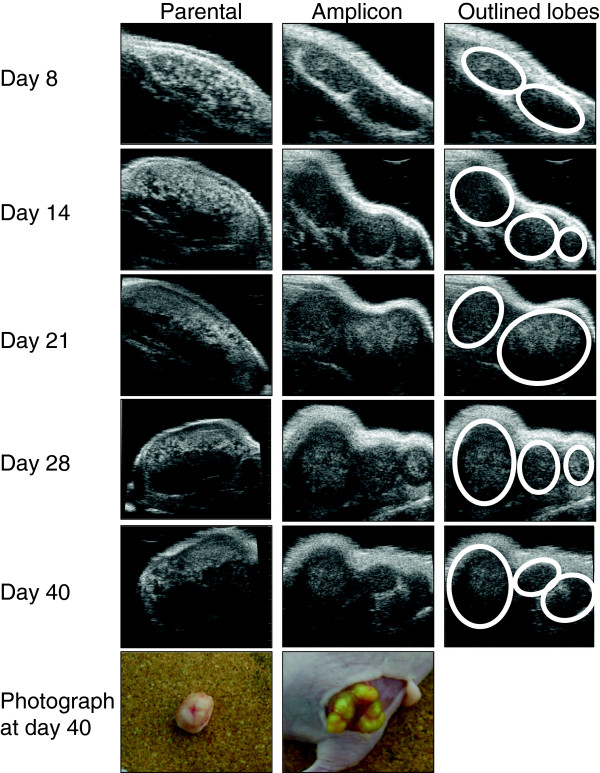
High-frequency ultrasound imaging showing anatomical detail of xenograft growth that corresponds with *ex vivo *examination. HF-US images of representative xenografts at the indicated day of growth. The first column shows images from an uninfected xenograft (parental cell line). Note that by day 28 the imaging plane was changed to be able to fit the xenograft into the field of view and scan over the whole tumour to generate the 3D image. The second column shows the amplicon infected xenograft. The third column outlines the lobes visible in the amplicon xenograft. The photographs in the column are of each xenograft at day 40 showing the distinct lobes of the amplicon infected xenograft compared to the uninfected xenograft.
